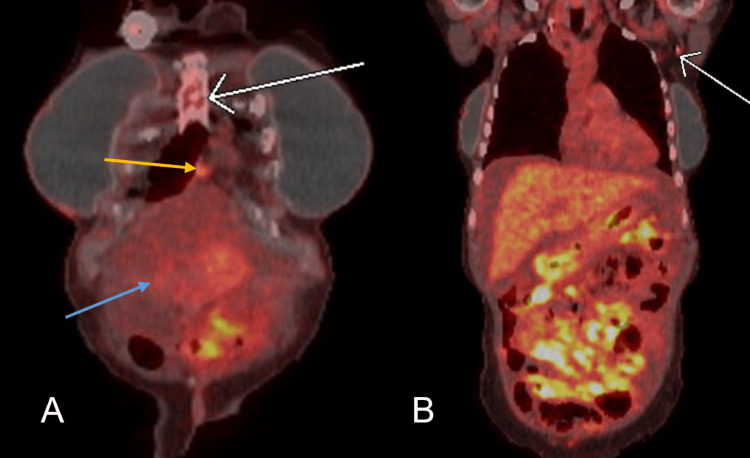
(A) Coronal view from positron emission tomography-computed tomography (PET-CT) showing worsening hypermetabolic retrosternal (white arrow) and pericardial (yellow arrow) lymph nodes. Also showing an interval increase in mildly metabolic ascites (blue arrow). (B) Coronal view from PET-CT showing new hypermetabolic activity in the left axillary lymph nodes (white arrow).
